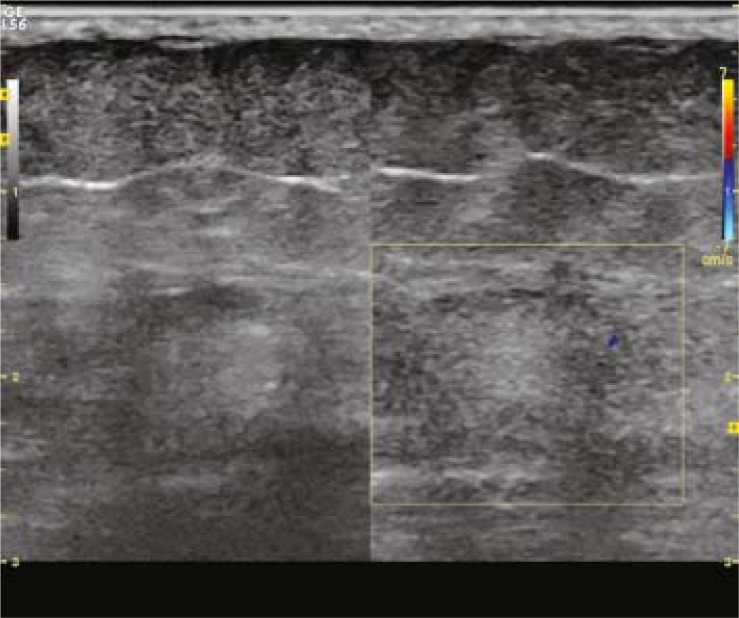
Pelvic colour Doppler ultrasound shows a lesion peripherally hypoechoic and hyperechoic in the middle with a diameter in 10 mm in the right gluteal adipose tissue. A clear blood supply example was not observed.
